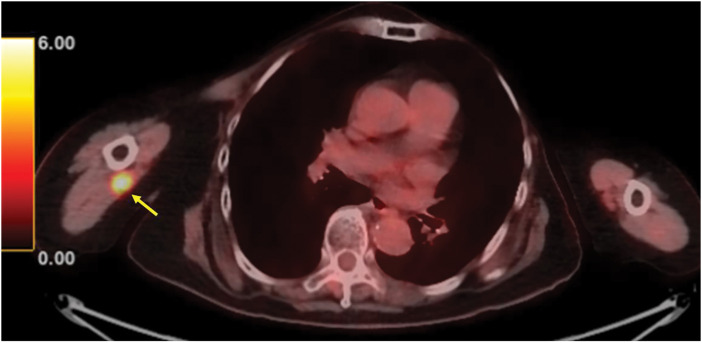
PET-CT axial and coronal images showing an FDG-avid lesion. PET-CT, positron emission tomography-computed tomography; FDG, fluorodeoxyglucose
